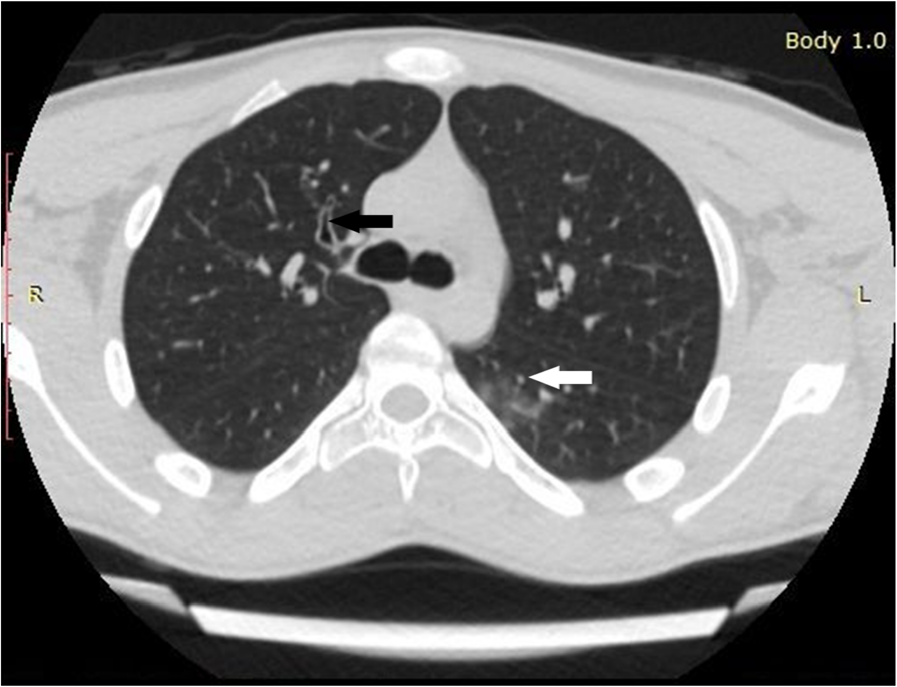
Axial non-contrast enhanced chest CT of 14-year-old boy, 1 day after the onset of fever and running nose, there is unilateral GGO in the superior segment of the LT lower lung lobe (white arrow) and bronchial wall thickening in the anterior segment of the RT upper bronchus (black arrow)
